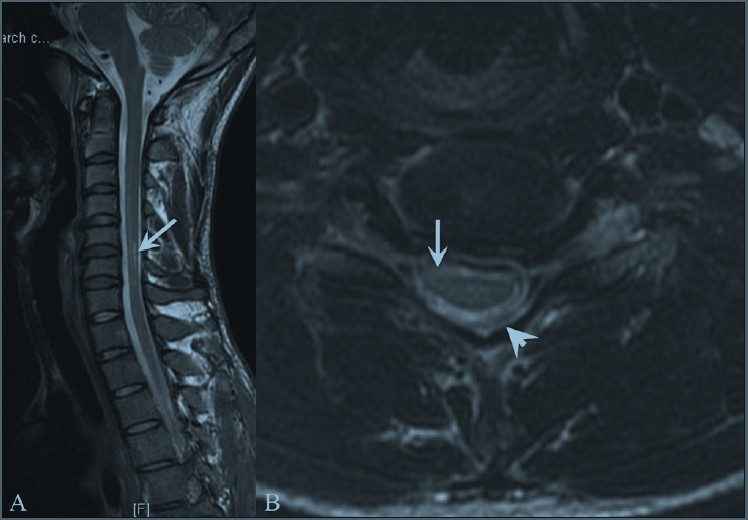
Neutral position T2W sagittal (A) and T2W axial (B) magnetic resonance images show localized cervical cord atrophy at the C5-C7 levels (arrows), which is asymmetrical on the axial image (arrow) with a pear-shaped cross-sectional appearance, with straightening of the cervical curvature and loss of attachment of the dorsal dura (arrowhead)
